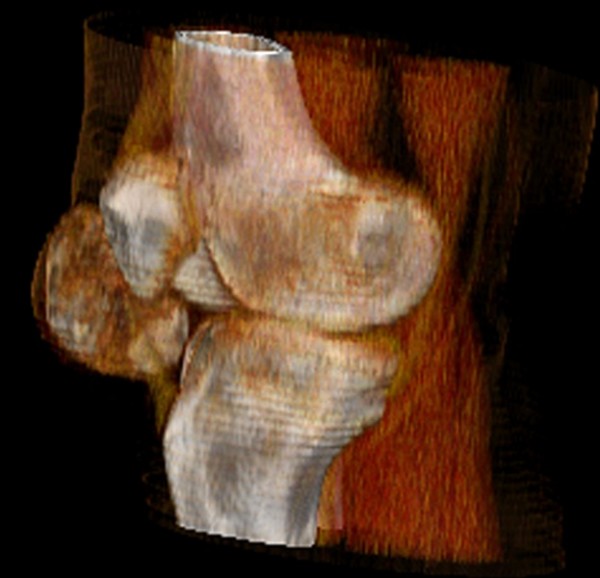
Volume rendering reformatted CT image shows the position of the osteochondral lesion with respect to patella, tibia and patellar tendon. The mass is not attached to adjacent bones.
